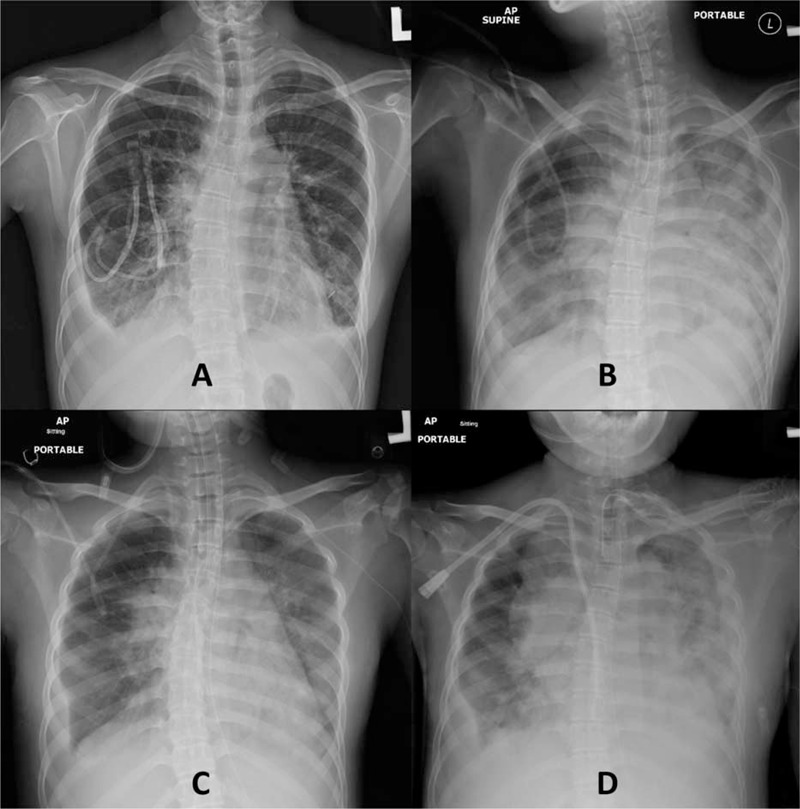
The serial chest x-rays perihaploidentical transplantation showing the following: (A) the appearance of bilateral pleural and pericardial effusions just after conditioning (day −1); (B) the rapid development of bilateral diffuse pulmonary infiltrates after engraftment (day +44); (C) the resolution of the pulmonary infiltrates after treatment with methylprednisolone and etanercept (day +50); (D) rapid tumor progression, including a mass in the neck, supraclavicular, axilla, mediastinum, lungs and pleura (day +83).
